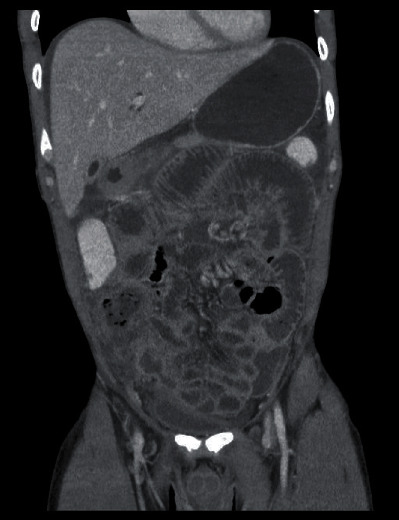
CT abdomen with evidence of ileus most localized in the area of the ileocecal valve with questionable characteristics. No evidence of intra-abdominal free air or peri/subhepatic ascites.
